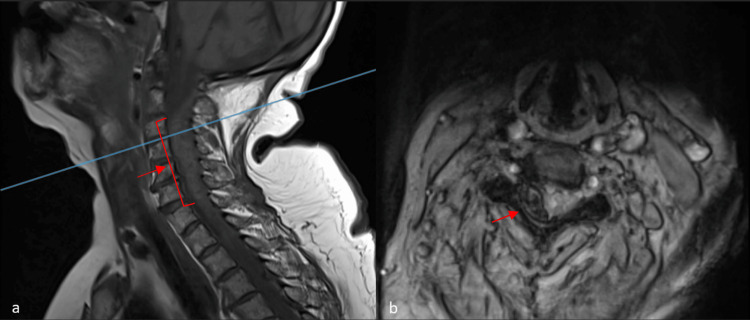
(A) T1-weighted MRI (sagittal view) showing a hyperintense collection from the C3 level down to the lower border of C7. (B) T2-weighted MRI (axial view) at the C3-4 level, where the hematoma is most prominent, showing complete obliteration of the exiting right nerve root.
